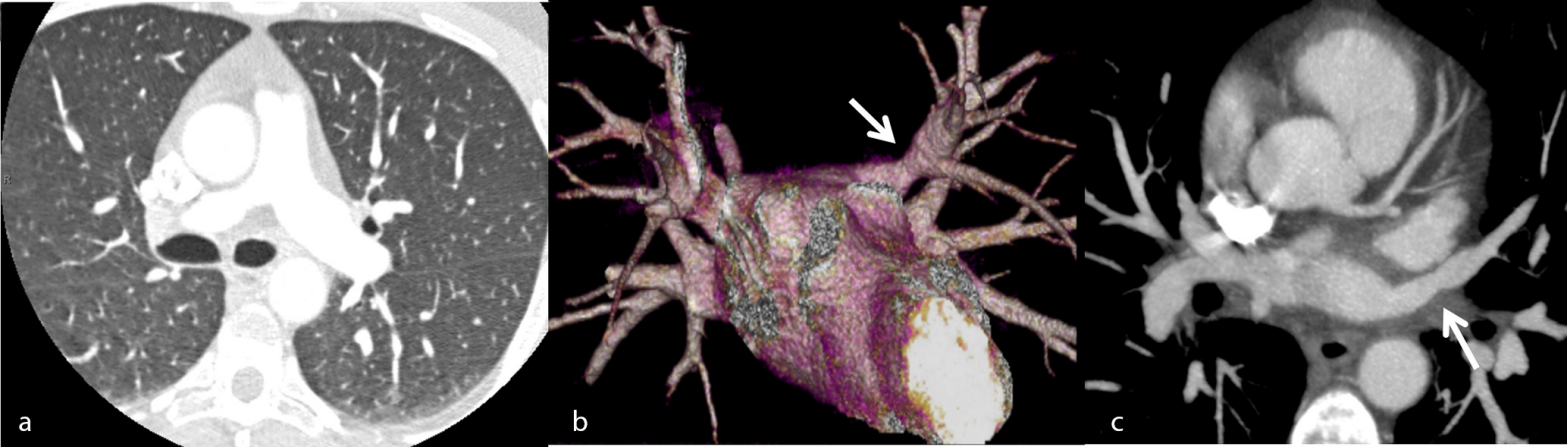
Three months post-ablation follow-up cardiac CT scan: (a) Lung window shows normal pulmonary parenchyma. (b and c) 3D reconstruction of left atrium and mediastinal window enhanced CT axial section respectively show left upper pulmonary vein LSPV moderate stenosis (arrows).
